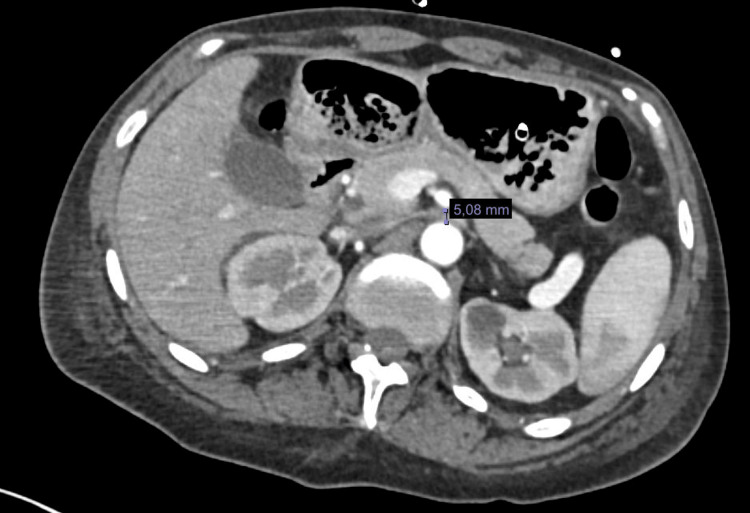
Axial CT scan. Measurement of aortomesenteric distance (distance of 5.08 mm). CT: computed tomography
